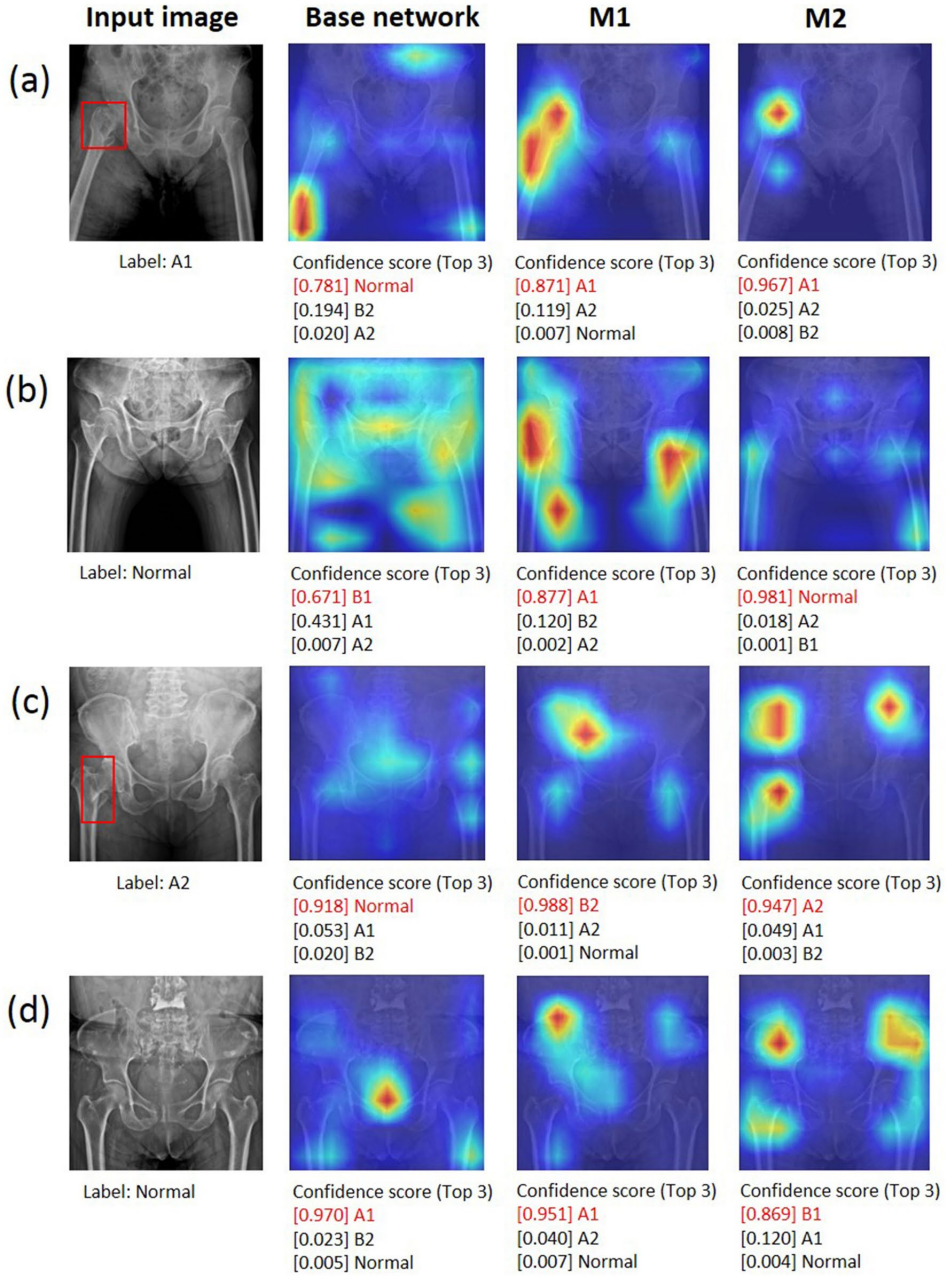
Examples of Grad-CAM-assisted images with the three models. (a) For the pelvic radiograph labeled as A1, all models were predicted correctly. (b) For the first normal image, only M2 was predicted correctly. (c) For the fracture image labeled A2, only M2 was predicted correctly. (d) For the second normal image all models were predicted incorrectly. The red boxes are fracture lesions.
